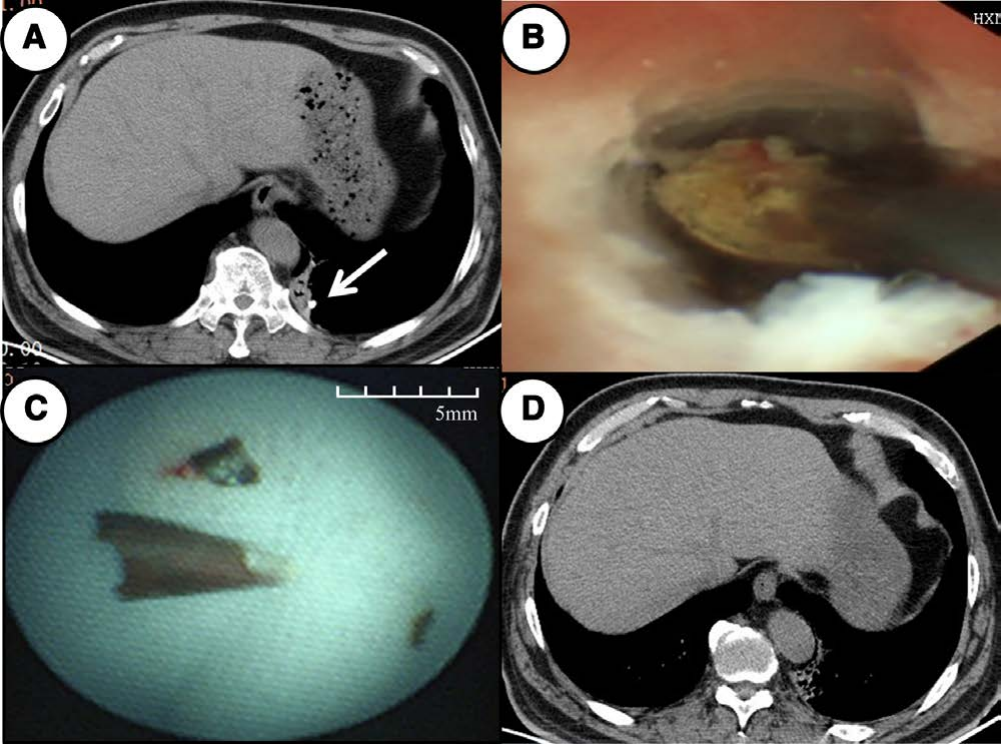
(A) Reexamined CT images indicated that the foreign body was pushed further away (arrow). (B) The cryoprobe was inserted into ultrathin bronchoscope (UTB), keeping the probe against the foreign body, and freezing. (C) The remaining chili was completely extracted. (D) Reexamined CT images confirmed the completely removal of the foreign body.
